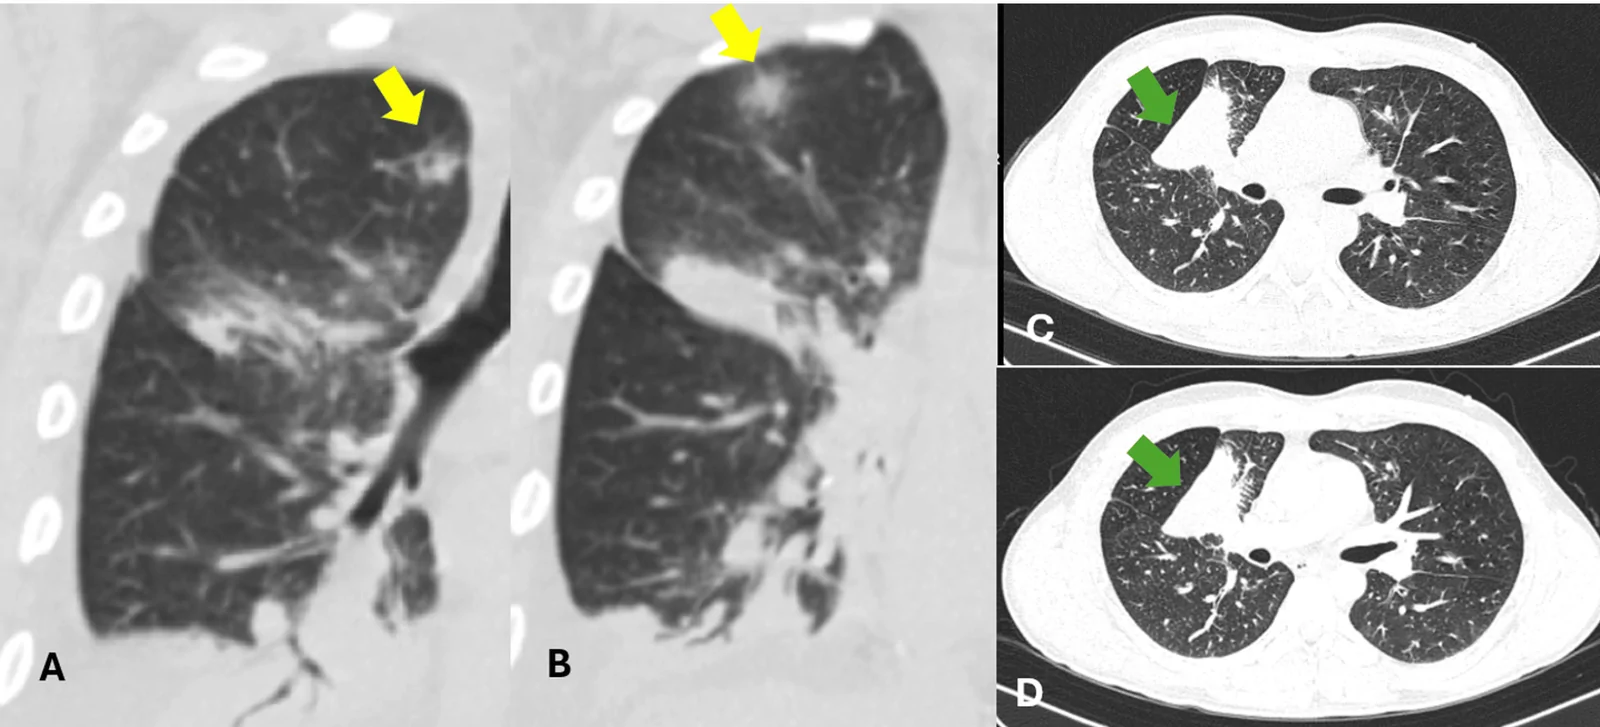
Chest computed tomography (CT) A–B (coronal reconstructions): Peripheral and subpleural ground-glass opacities are identified in the right upper lobe, with areas of mild confluence (yellow arrows). C (axial image at admission): Alveolar consolidation in the anterior segment of the right upper lobe, with ill-defined margins (green arrow). D (axial image after 14 days of treatment): Persistent alveolar opacity in the same region, with minimal change in extent and density, indicating limited radiological response to therapy (green arrow).
